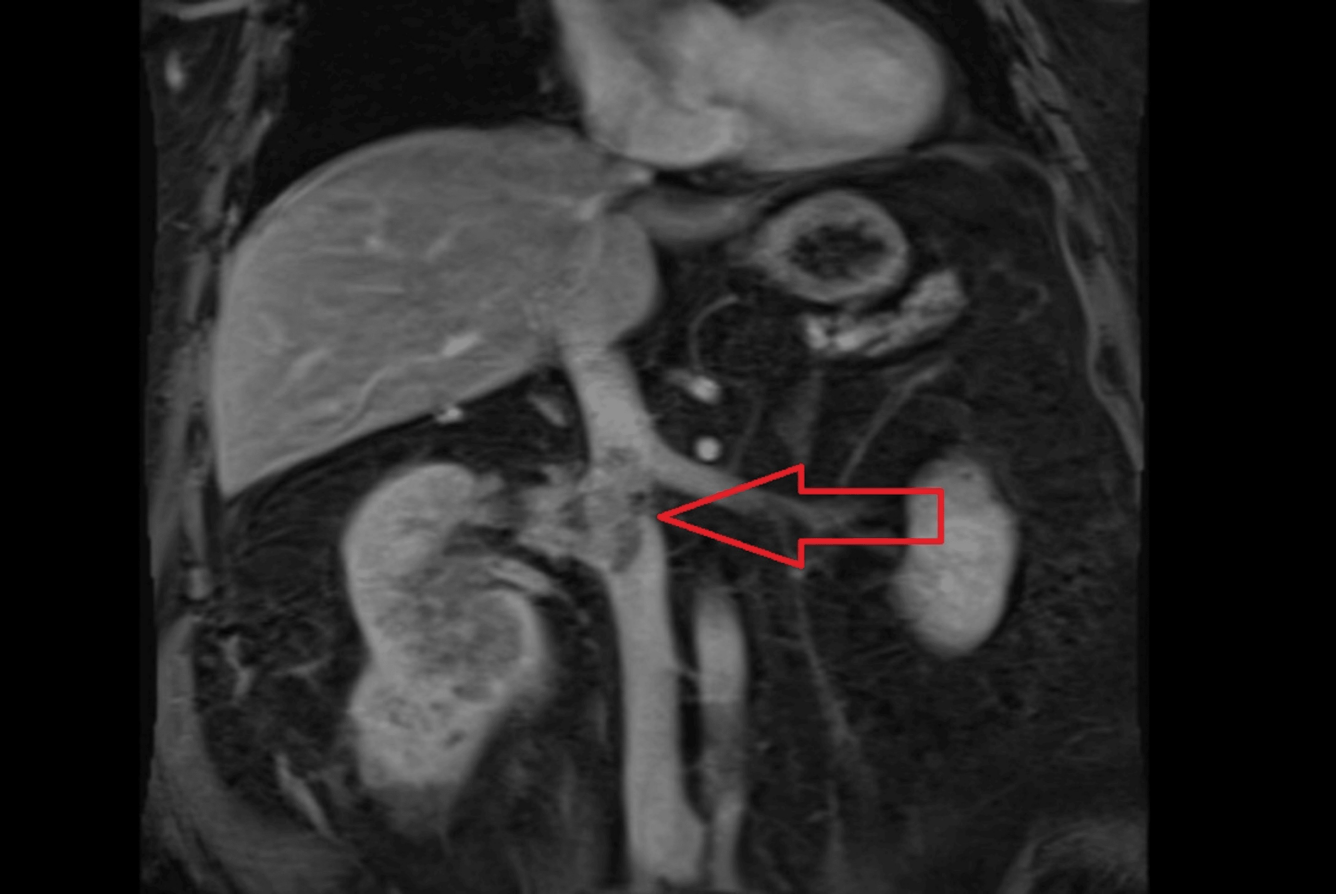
MRI Image Showing IVC Tumor Thrombus IVC: Inferior vena cava
